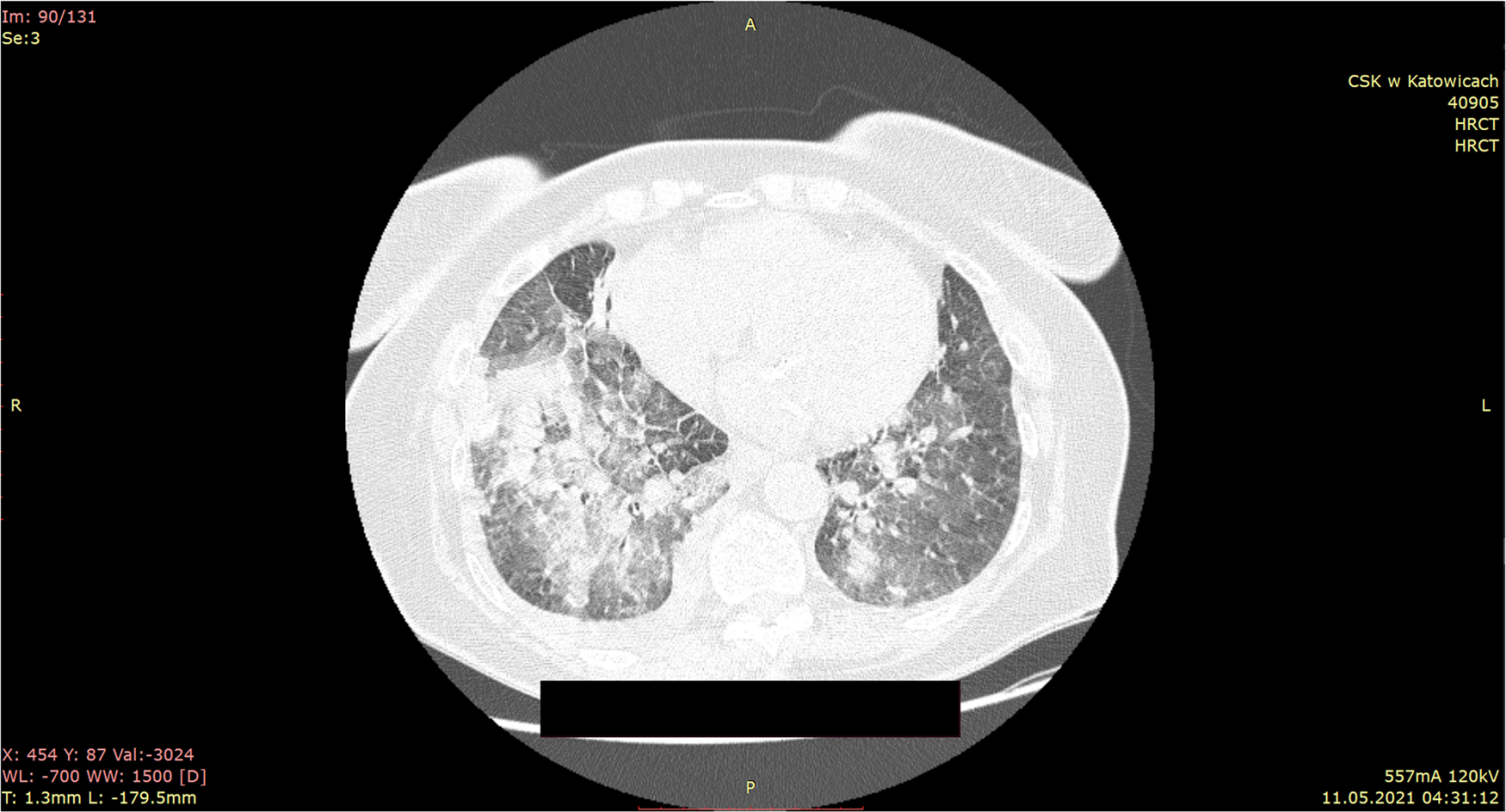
HRCT scans on hospital admission: bilateral, superimposed air space consolidations with GGO in lower and upper lobes, more marked on right are described.
